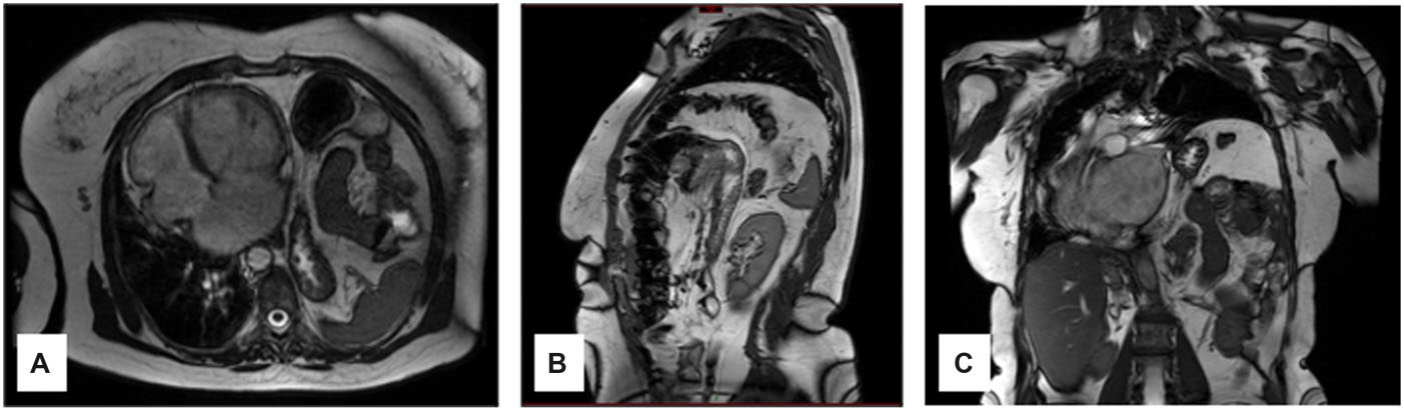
Preoperative dynamic diaphragmatic dynamic MRI (*sequence Fiesta gradient echo steady state GE Architect 3 T 2019)*. (A) Axial view showing the mediastinal shift with major left atrial and ventricular dilatation. (B) Sagittal view of the major left diaphragmatic eventration. (C) Coronal view of a mediastinal shift.
